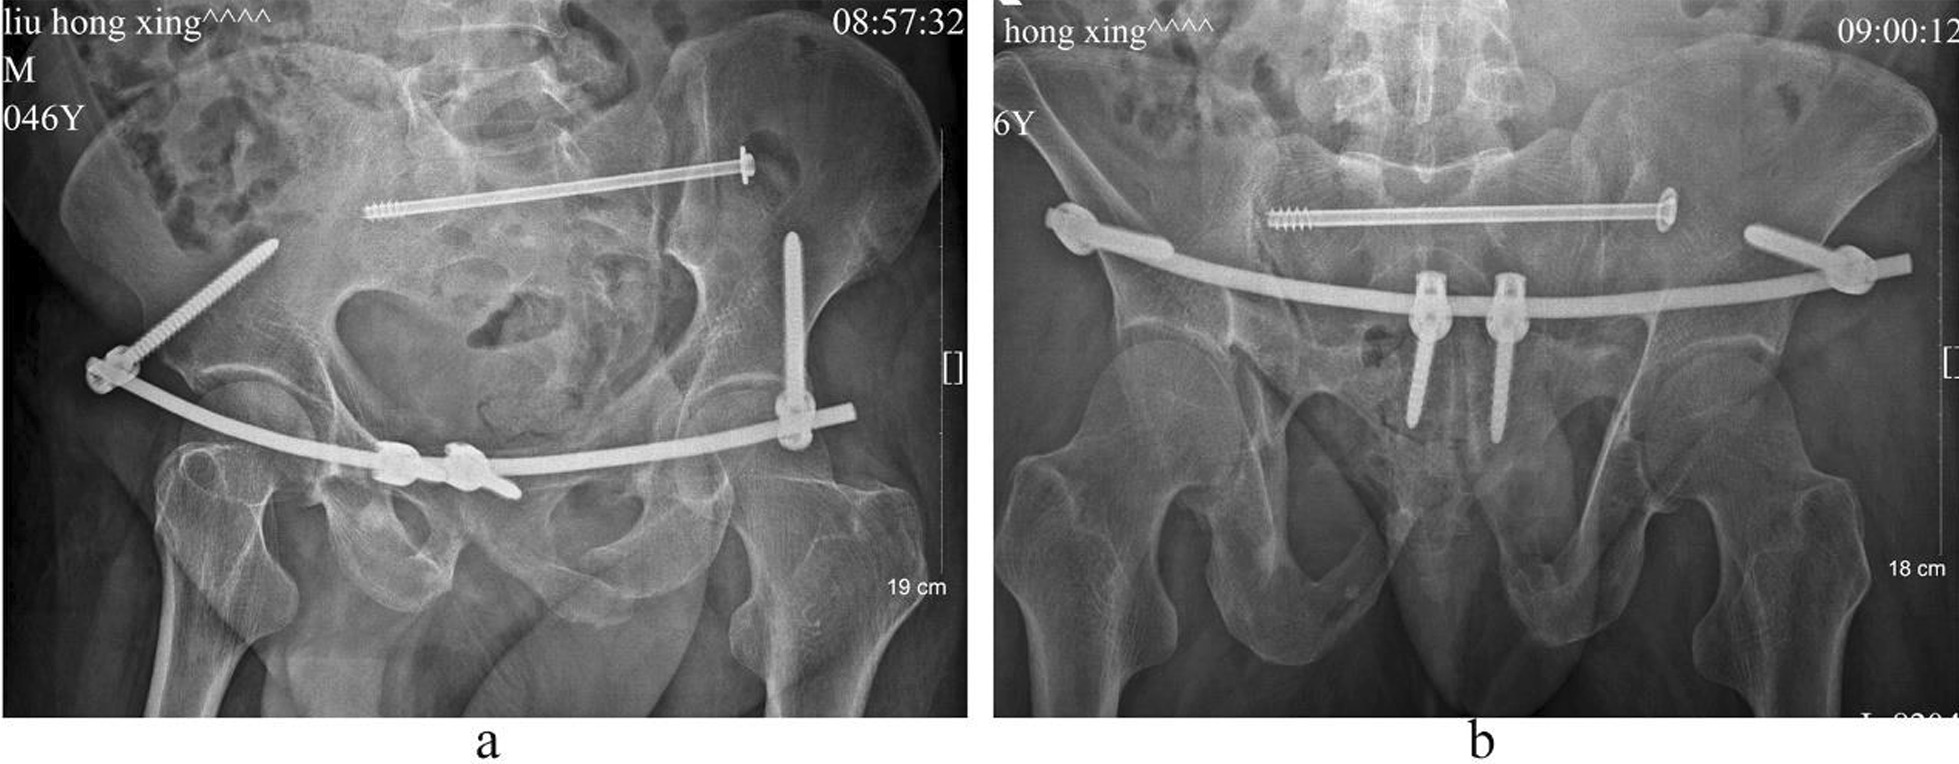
Postoperative X-ray images of case 3. (a Pelvic anteroposterior X-ray image, b pelvic outlet X-ray image.)
